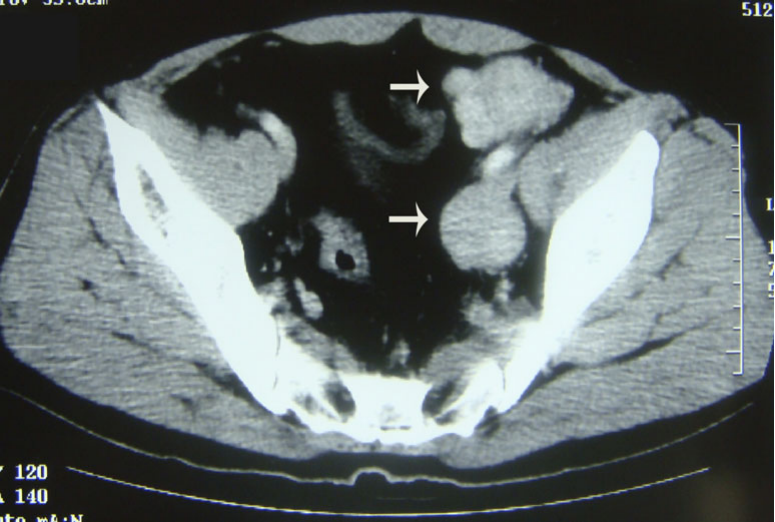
CT scan showing smaller masses located in the pelvis cavity.
